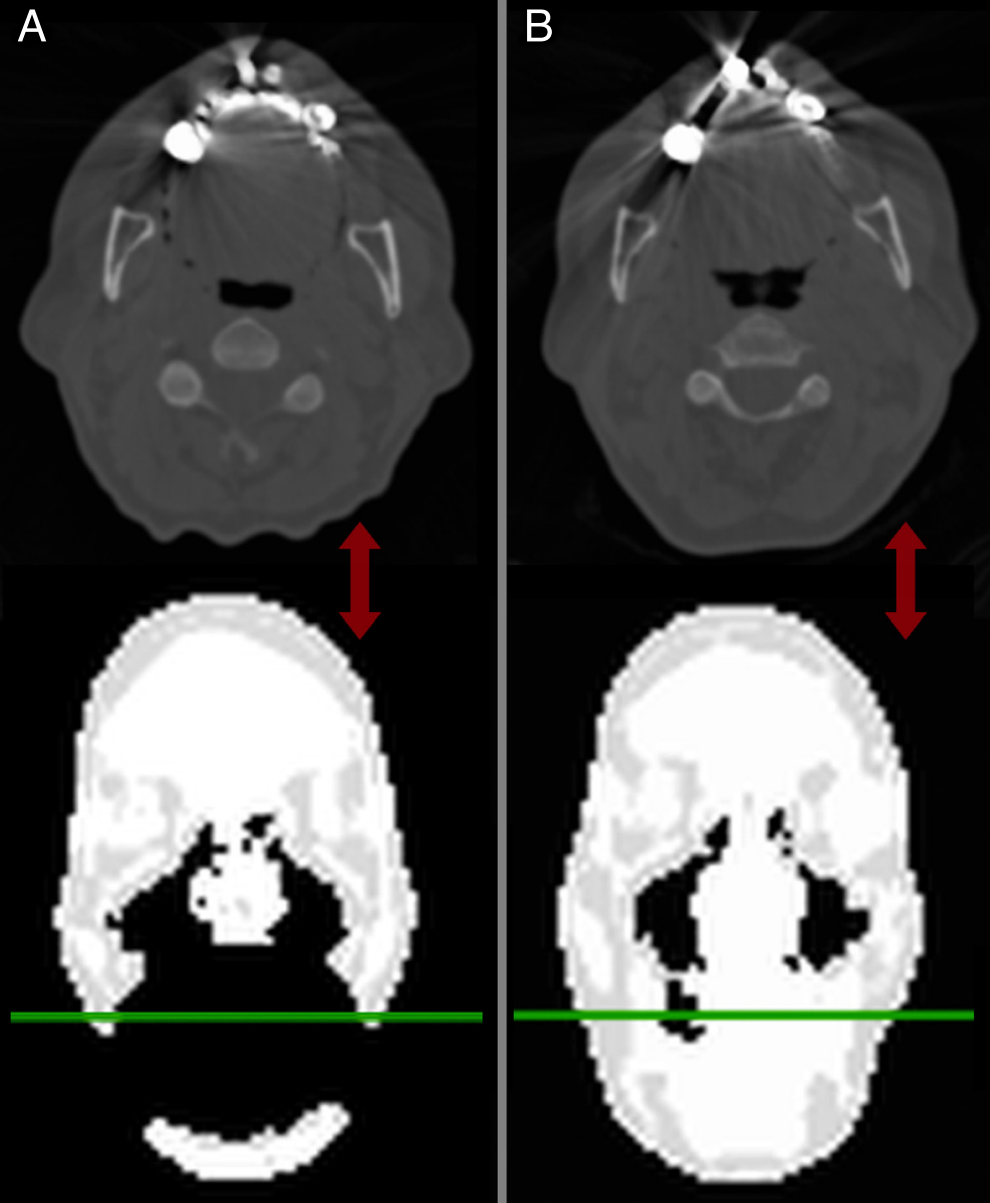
Demonstration of the irreproducibility of the artifact sizes between scans. MR-AC (bottom row) compared to CT (upper row). (A,B) MR-AC_DIXON_ and CT images of the same patient acquired 4 months apart. Note that the magnitude and size of CT artifacts seem unchanged between the scans.
